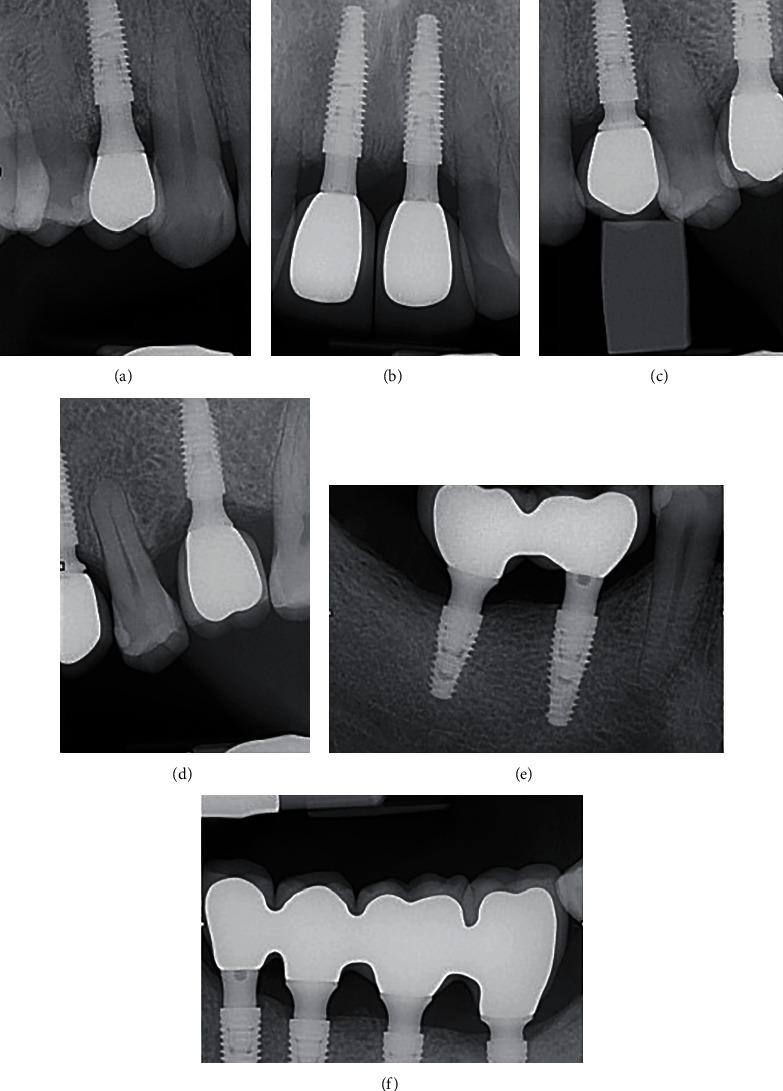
Periapical X-rays after maxillary (a)–(d) and mandibular (e, f) definitive prosthesis installation.
